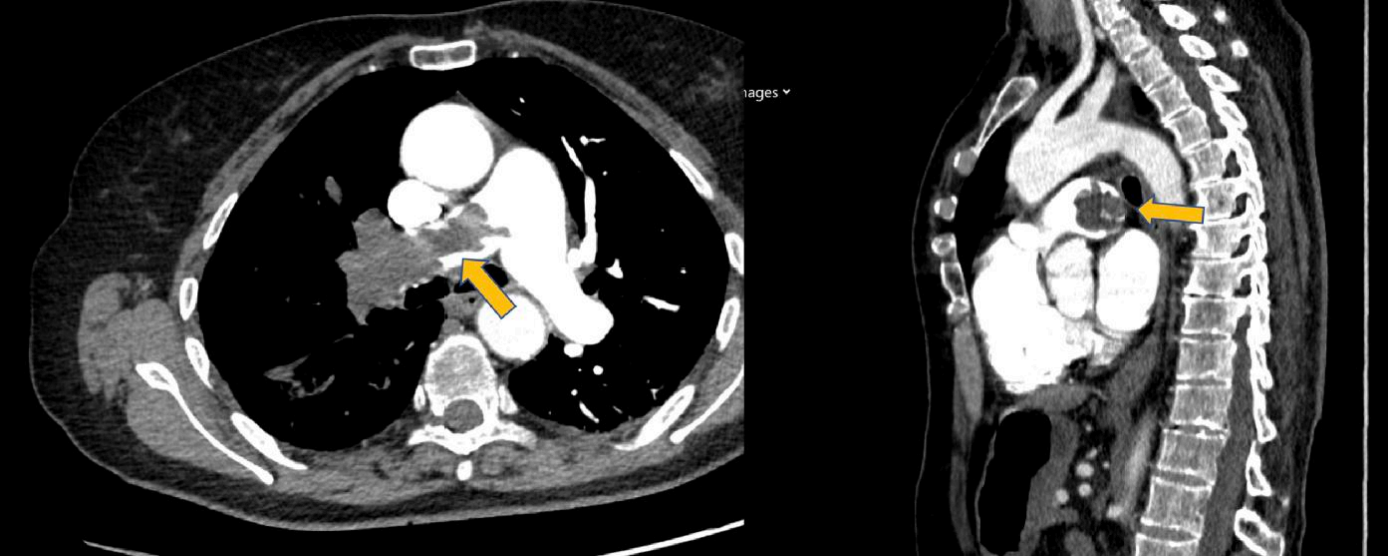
Computed tomography scan showing the pulmonary angiosarcoma, indicated with the yellow arrows.
